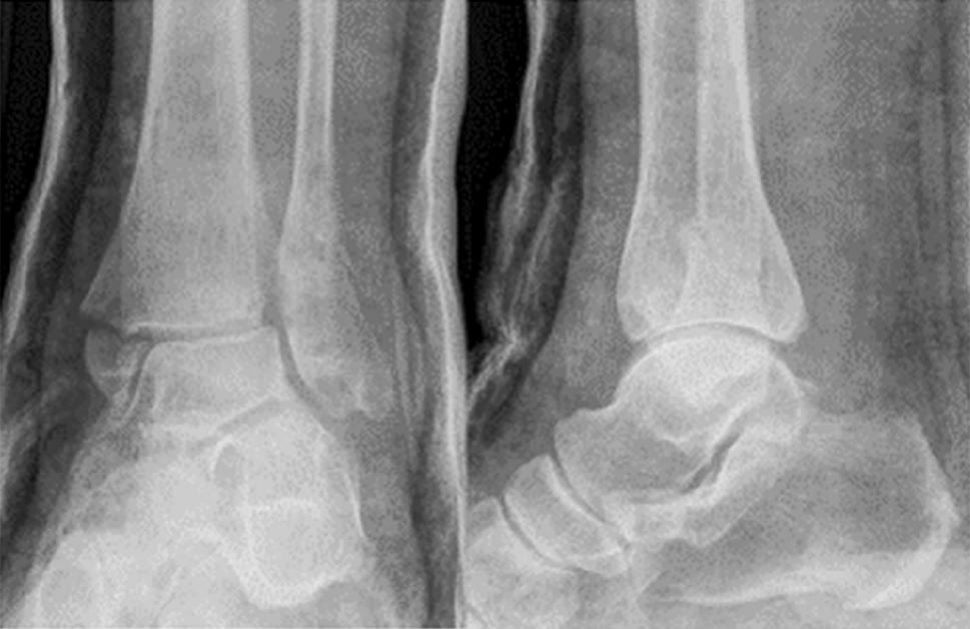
Anteroposterior and lateral radiographs of a 60 year old female patient with a pronation-abduction injury to her left ankle following cast application
